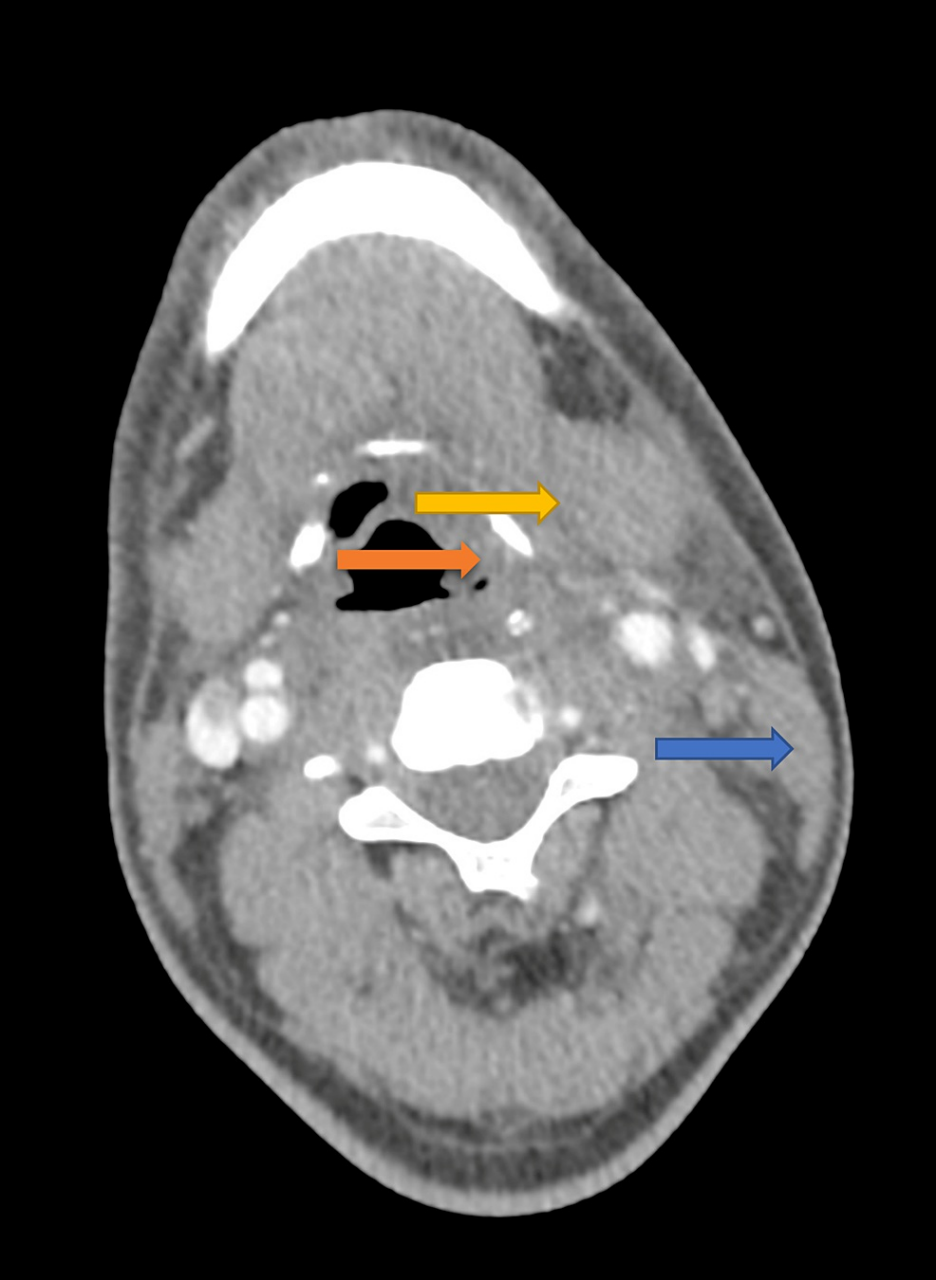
CECT image of neck/thorax Contrast-enhanced computed tomography (CECT) neck/thorax showing left aryepiglottic fold asymmetrically bulky (shown in orange arrow), left sternocleidomastoid muscle appears bulky and edematous (shown in blue arrow), and obliteration of the left vallecula (shown in yellow arrow).
